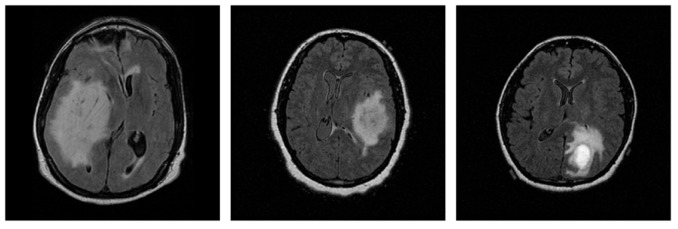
Representative 2D MRI slices of astrocytomas from each grade in the dataset: Grade 2 (left), Grade 3 (center), and Grade 4 (right).
